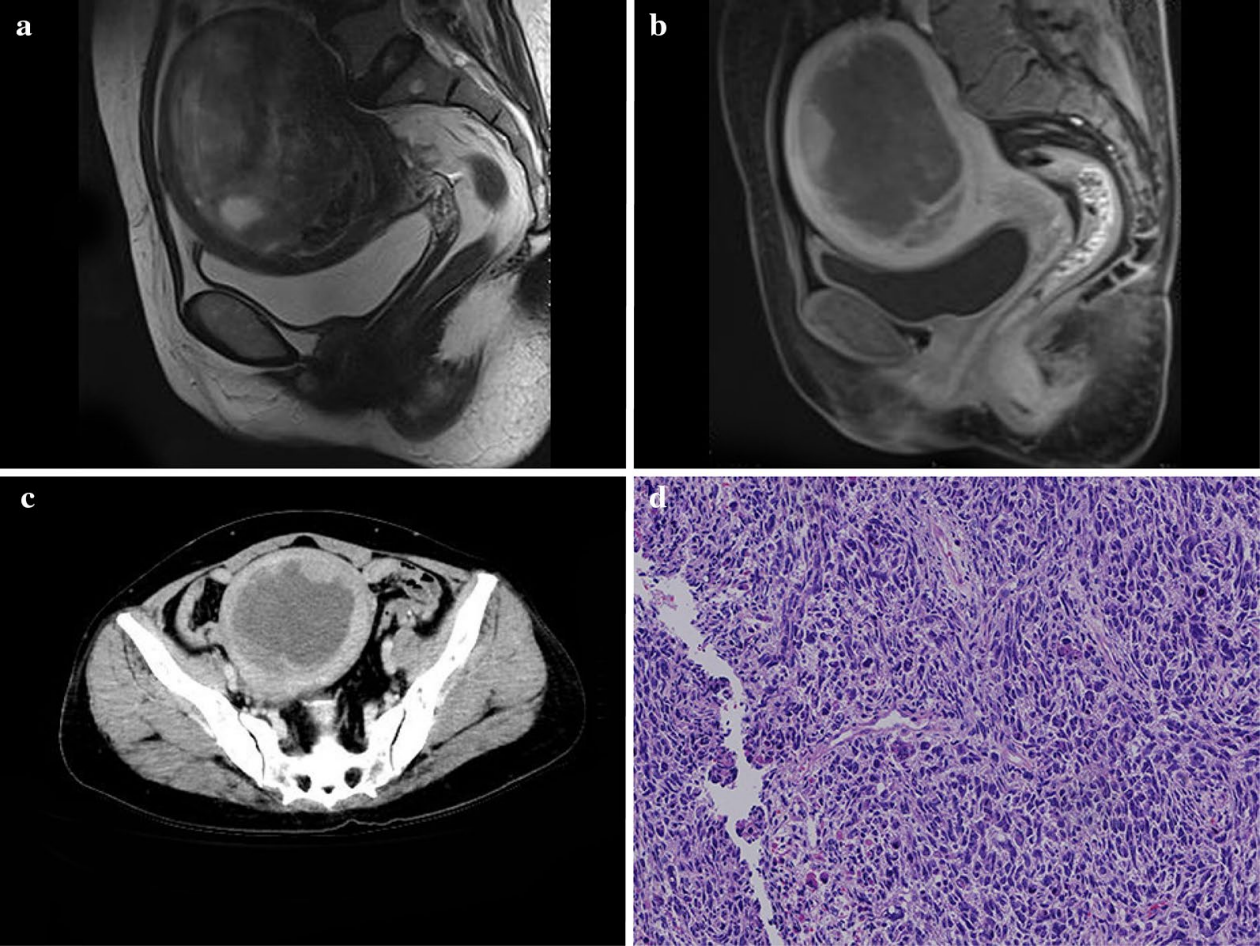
A 53-year-old woman with uterine carcinosarcoma (UCS). Sagittal T2WI magnetic resonance imaging (MRI) (a) showed a mixed signal tumor in the cavity complicated with intratumoral hemorrhage. Sagittal delayed enhanced T1WI (b) and axial computed tomography (CT) images in the venous phase (c) showed the marginal solid tissue component polyp-like enhancement with a central cystic component. The pathological image (d) showed uterine carcinosarcoma
